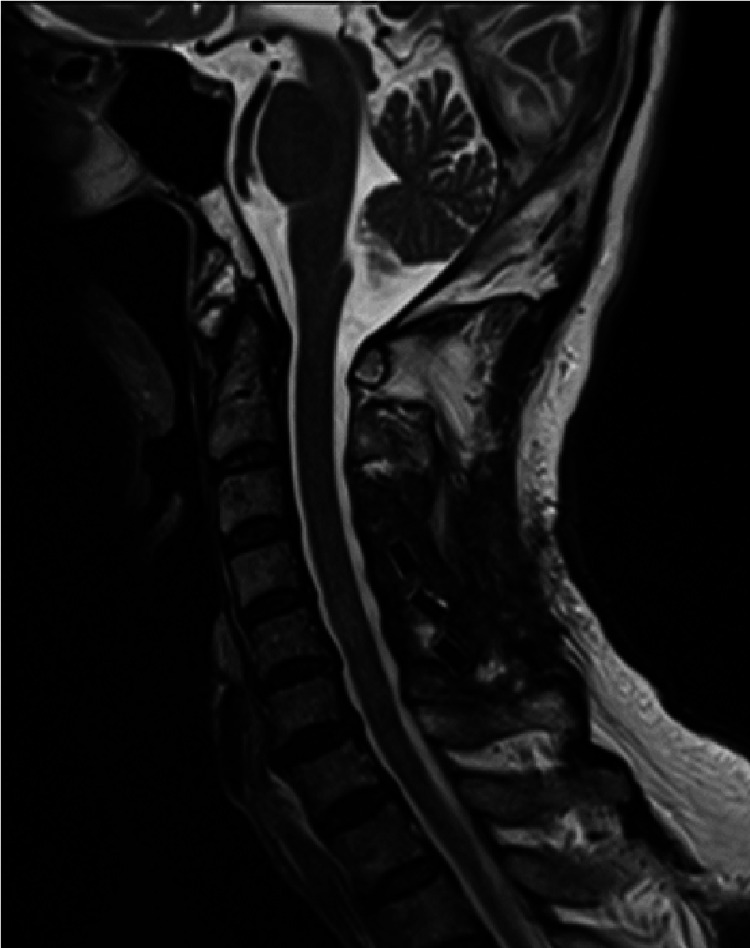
Case 2 postoperative cervical sagittal T2-weighted MRI image
